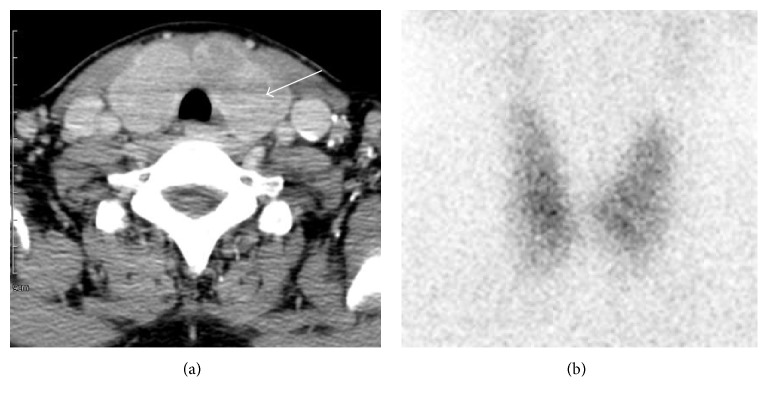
(a) Neck computed tomography shows 1.9 × 1.2 cm sized hypodense mass involving thyroid isthmus in the left thyroid. (b) Thyroid scan with Tc-^99^m pertechnetate shows diffuse and even uptake in both thyroid glands.
